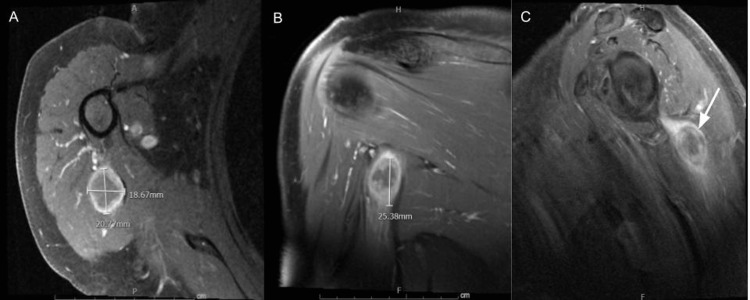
MRI of the right shoulder showed an enhanced solid mass (white arrow) within the proximal long head of the right tricep muscle (A: axial view, B: coronal view, C: sagittal view).
